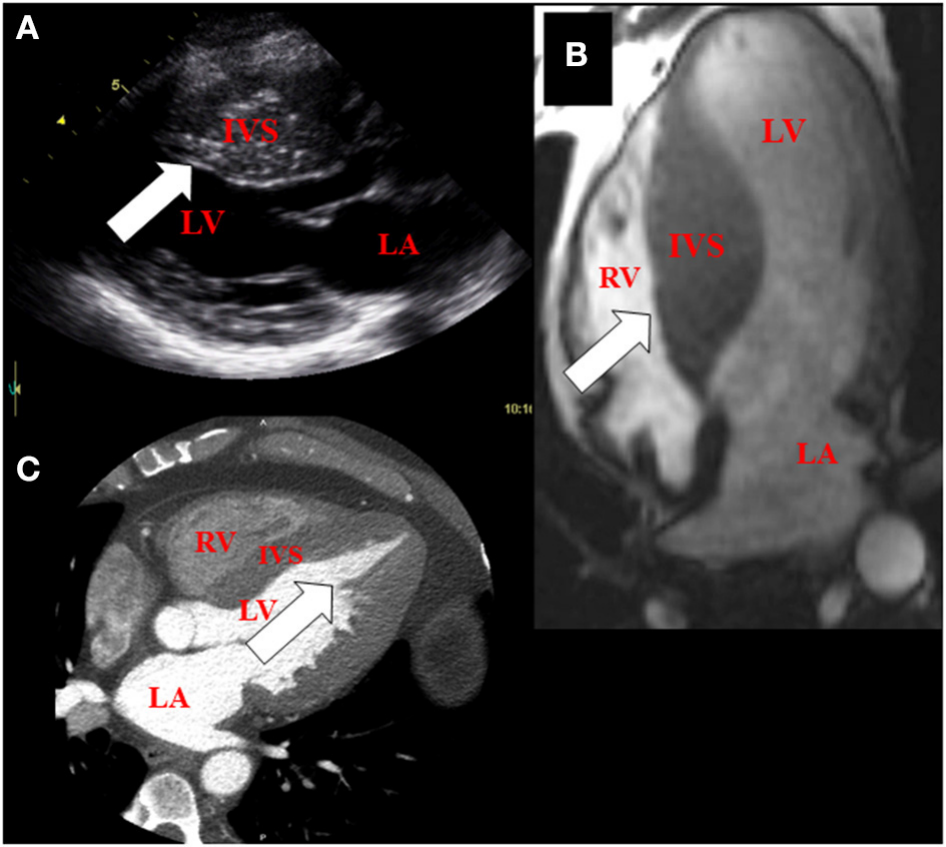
Imaging features of hypertrophic cardiomyopathy using multi-modality cardiac imaging in patients. (A) Basal hypertrophy of the inter-ventricular septum (arrow) using echocardiography (parasternal long axis view of the heart). (B) Mid-septal hypertrophy (arrow) using magnetic resonance imaging (4-chamber view of the heart). (C) Apical hypertrophy (arrow) using Computed tomography (4-chamber view of the heart). LA, left atrium; IVS, inter-ventricular septum; LV, left ventricle; RV, right ventricle.
